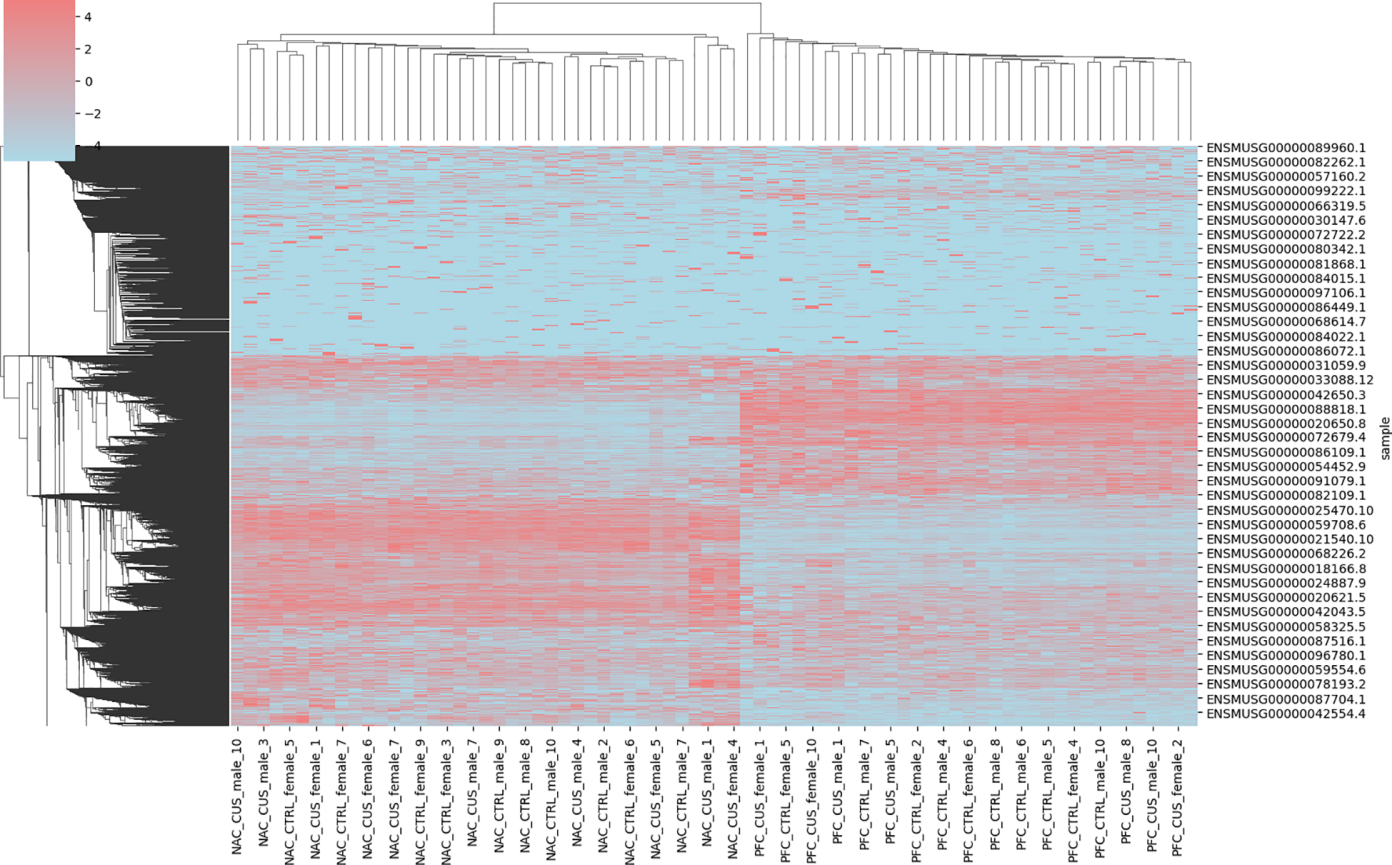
Visualization of the head and neck ultrasound dataset. Hierarchical clustering heatmap showing Z-score normalized expression values of differentially expressed genes across brain regions (NAC and PFC) and treatment conditions (CUS and control). Each row represents a gene (labeled by Ensembl ID), and each column corresponds to a sample. Samples are divided into four groups: NAC control (NAC_CTL), NAC CUS-treated (NAC_CUS), PFC control (PFC_CTL), and PFC CUS-treated (PFC_CUS), as indicated on the x-axis. Red and blue indicate upregulation and downregulation, respectively. The heatmap reveals distinct transcriptional signatures associated with both brain region and stress exposure.
